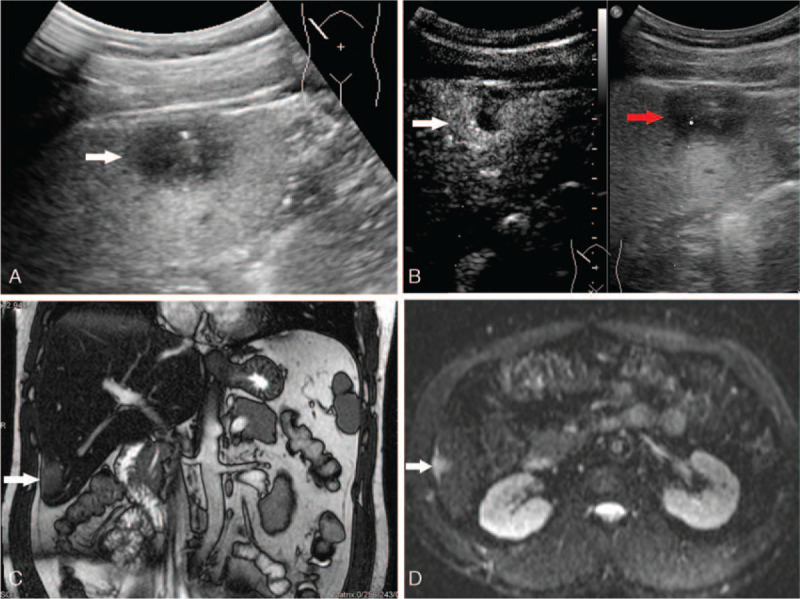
A: A hypo echoic lesion with a size of 2.5 cm x 1.8 cm located in the sixth segment of liver was detected on the transabdominal US. B: The lesion showed hyper enhancement on early arterial phase (white arrow) and quickly wash-out on late arterial phase (red arrow) after intravenous injection of sulfur hexafluoride-filled micro bubble contrast agent. C: A hyper intensity lesion with a size of 2.1 cm x 1.7 cm on T2-weight image of MRI was presented. D: The hyper intensity lesion was partly diffusion restricted on diffusion-weighted MR images.
